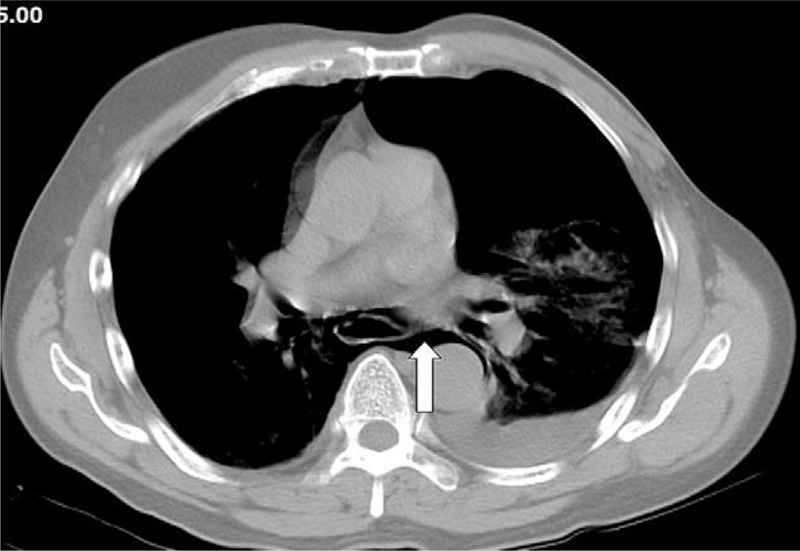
Contrast thoracic CT demonstrating massive extra-luminal air encompassing the peri-esophageal spaces (pneumomediastinum; arrow). CT = computerized tomography.
